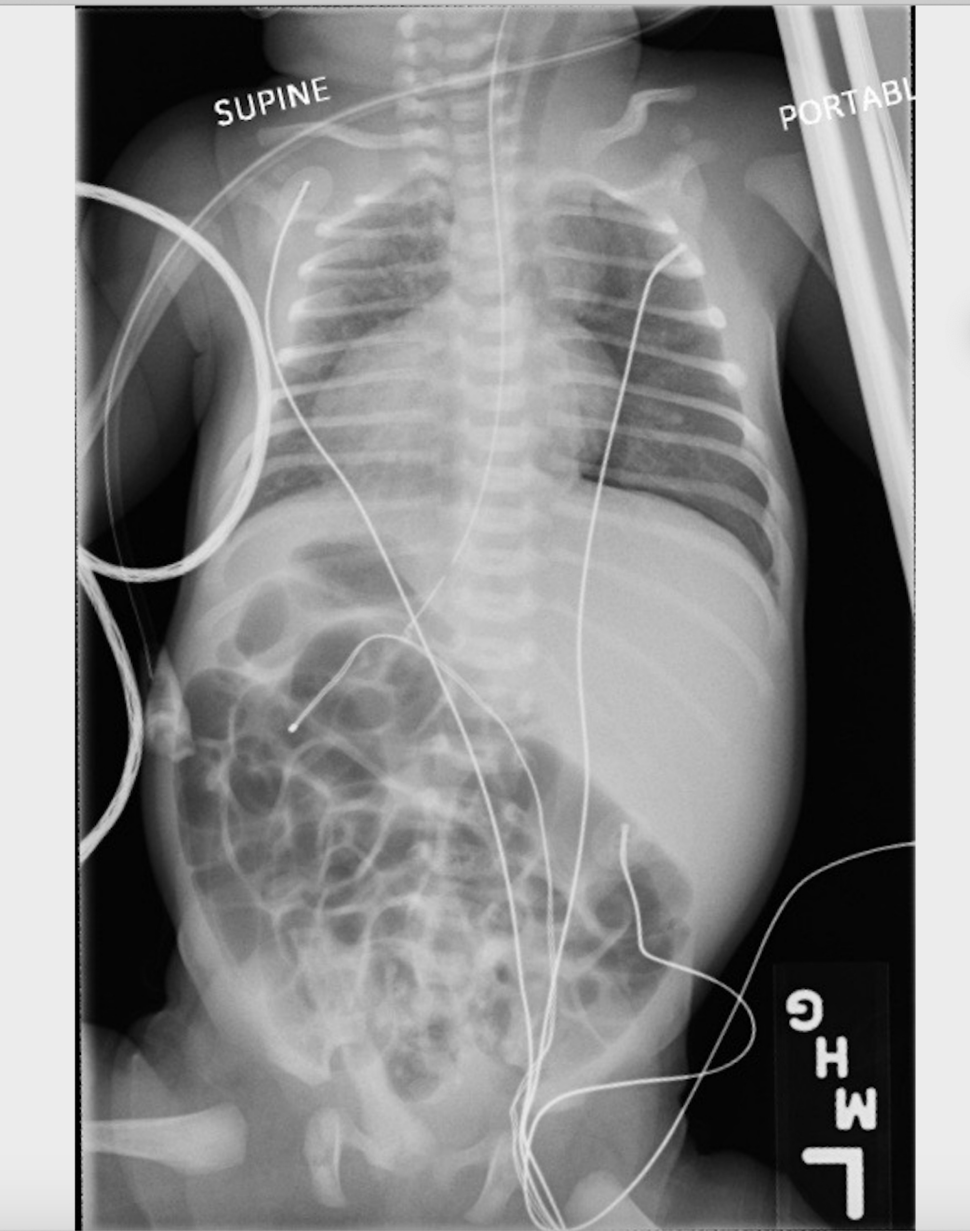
Chest X-ray of the infant suggesting situs inversus totalis
